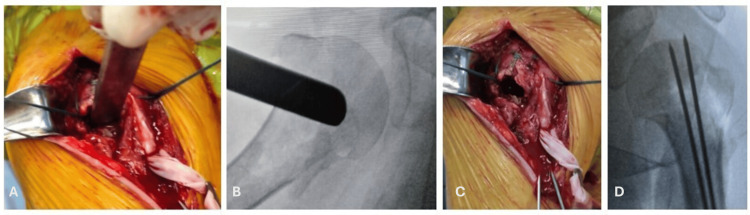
Intraoperative photographic image showing reduction maneuver of an impacted proximal humeral fracture (PHF) by articular surface elevation (A); intraoperative radiographic image showing fluoroscopy control of the reduction maneuver (B); intraoperative photographic image observing void formed beneath the humeral head (C) and intraoperative radiographic image observing PHF provisional fixation with two number two anterograde Kirschner wires (D).
